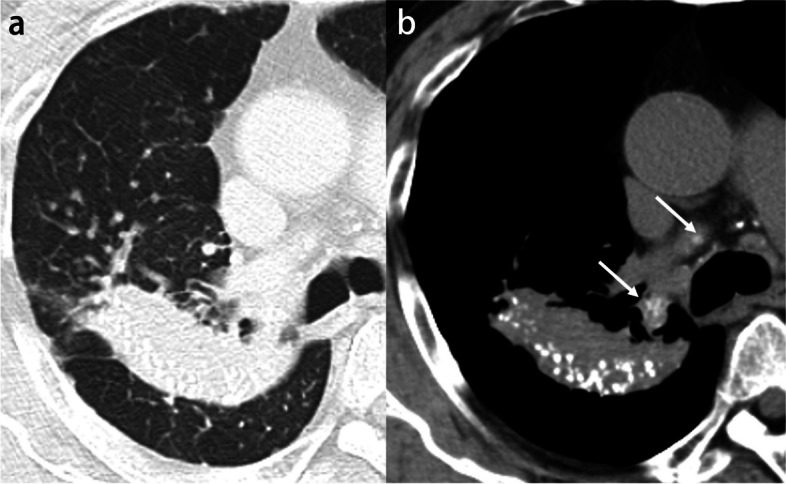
Silicosis (complicated form) in a 77-year-old man. a CT image shows a conglomerate mass, progressive massive fibrosis (PMF), in the right upper and lower lobes that crosses the major fissure. b Punctate and nodular calcifications are seen in the PMF. Mediastinal and hilar lymph nodes are also calcified (arrows)
